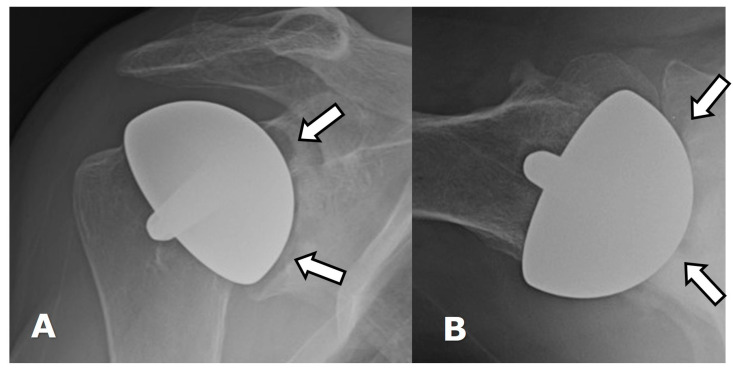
(A) Frontal and (B) axillary radiographs show glenoid wear with erosions (arrows) in a patient with an HHRA.
